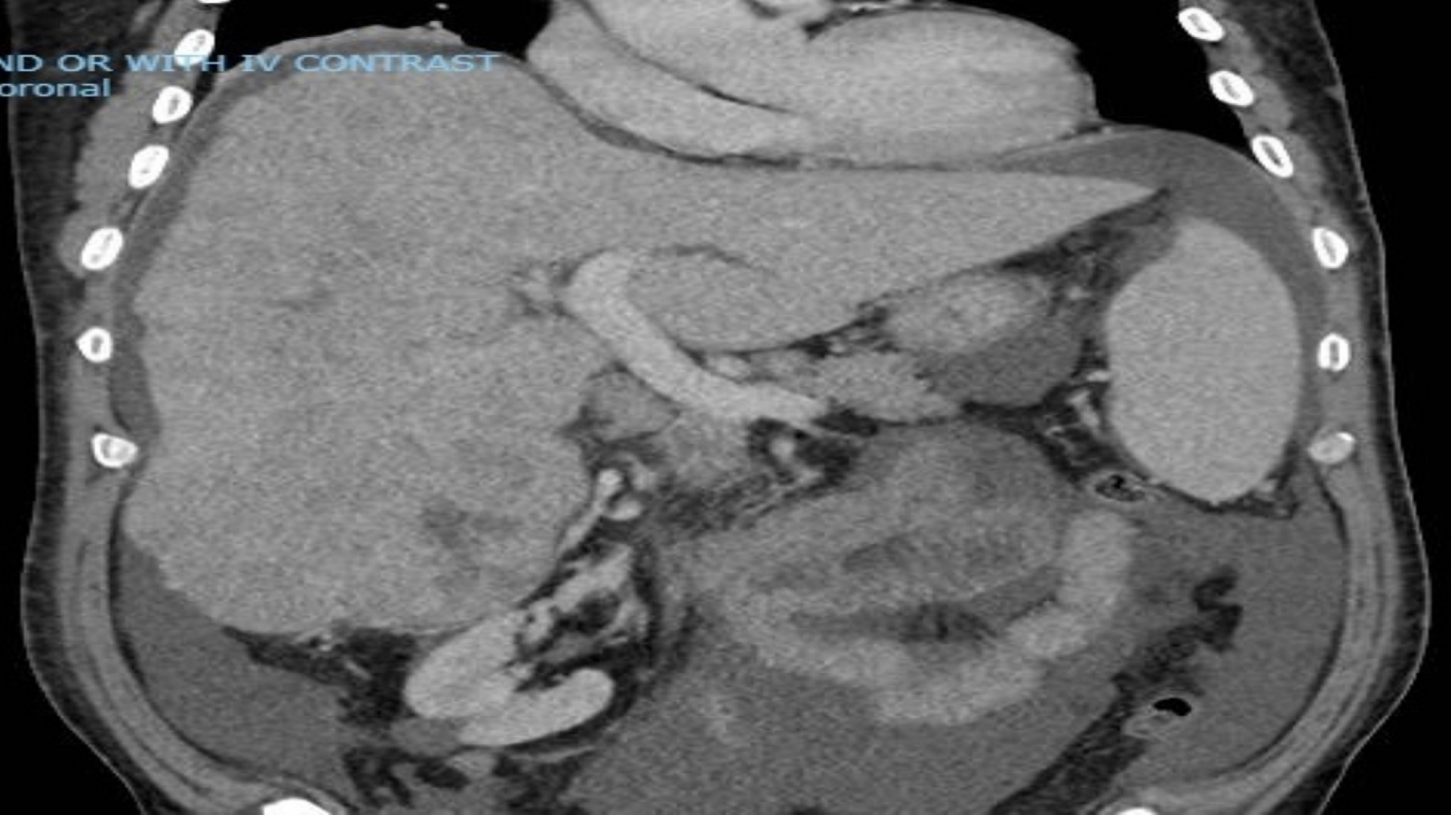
Computed tomography of the abdomen showing a cirrhotic liver with infiltrative tumor.
